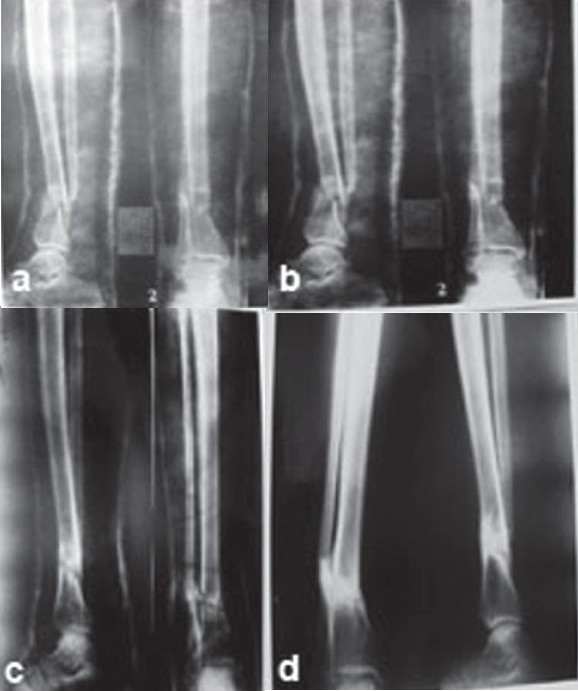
Sequential x-rays of leg bones (antero posterior and lateral views) of a 10 months old, initially compound fracture of distal one fourth of the bone of leg in a 34 year old male, at pretreatment stage (a); after 6 weeks of PEMS showing stage one healing (b); after 12 weeks PEMS showing stage and PEMS was discounted (c); 24 weeks after the star of PEMS showing established lamellar pattern and bone healing (d).
